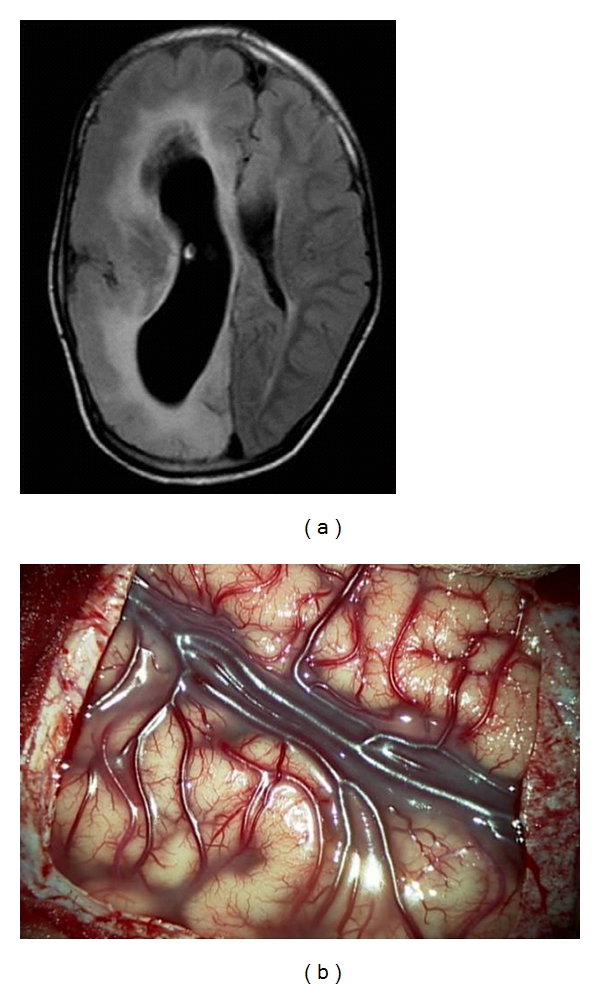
(a) T2W Axial Flair MRI Image of a patient with right hemimegalencephaly showing an enlarged right hemisphere with thickened cortical mantle, distorted ventricular anatomy, and widened gyri, (b) intraoperative photograph.
